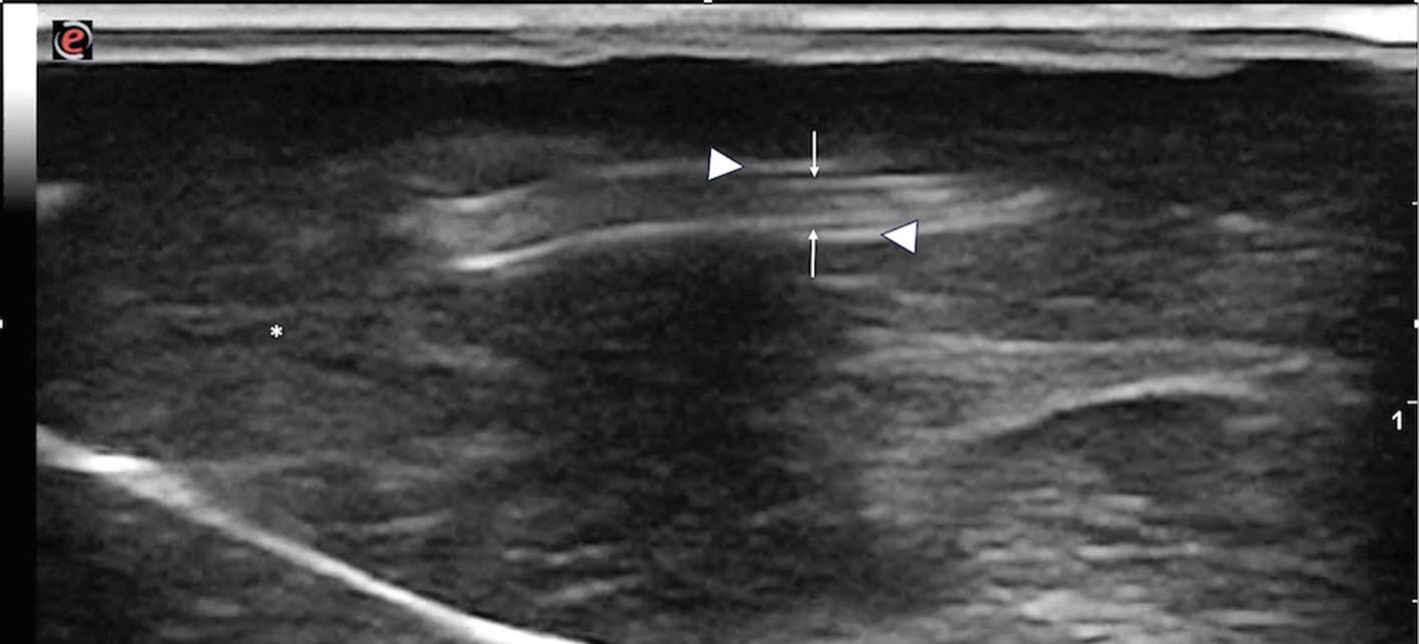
Ex vivo ultrasound image of the liver. The white arrows indicate a longitudinal parasite section, white arrowheads indicate the thickened walls of the bile ducts. *Liver parenchyma
